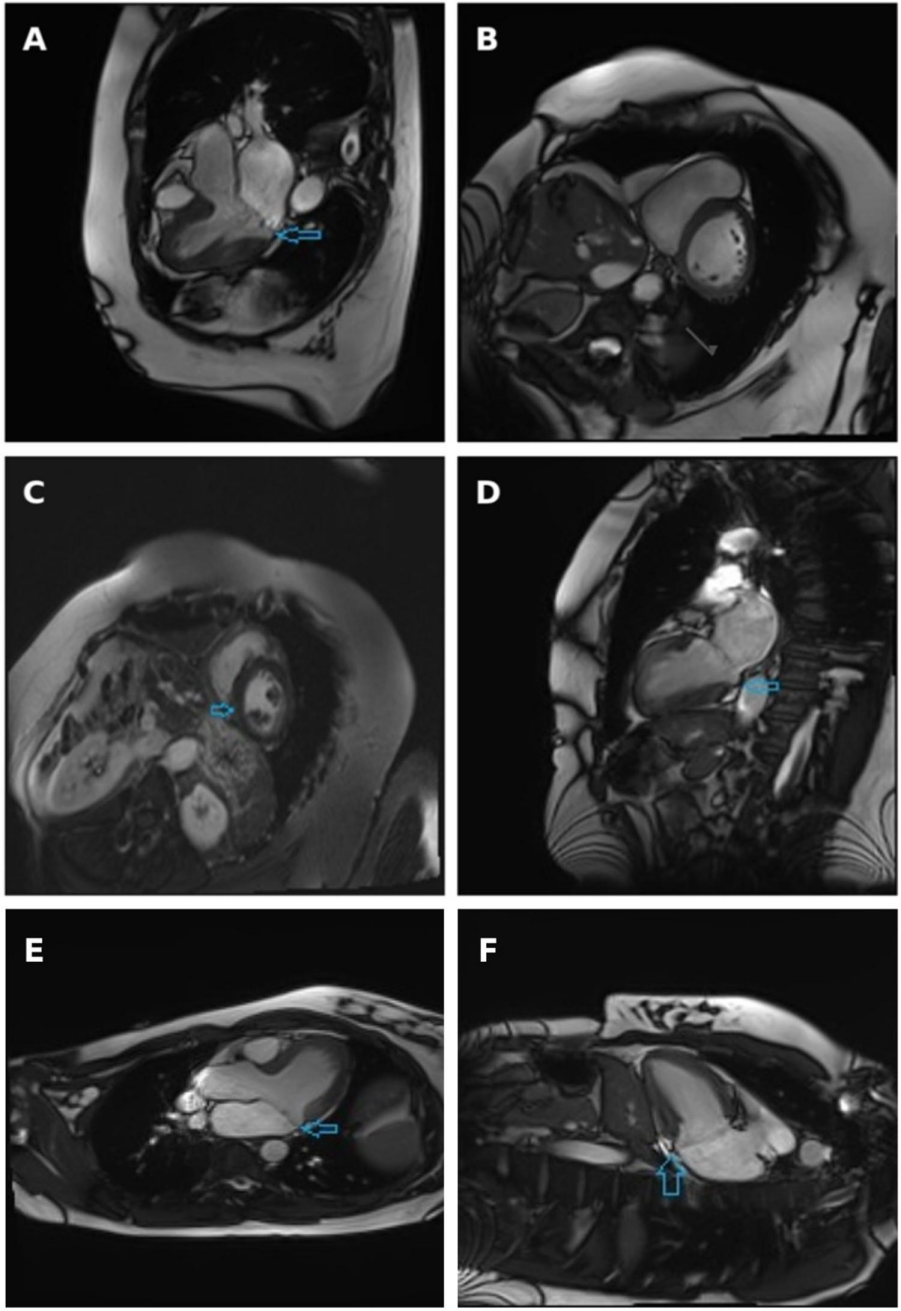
Cine three-chamber long-axis view (A), short-axis view at basal segments level (B) and two-chamber view (D) obtained at systole, with arrows indicating the separation between the posterior mitral valve leaflet insertion and the left ventricular myocardium. Late gadolinium enhancement short-axis view: arrow indicates non-specific fibrosis at the inferior right ventricular insertion point (C) Cardiac magnetic resonance imaging demonstrating mitral annular disjunction. Three-chamber long-axis view and (E) two-chamber view (F) obtained at end-systole, with arrows indicating the separation between the posterior mitral valve leaflet insertion point and the left ventricular myocardium.
